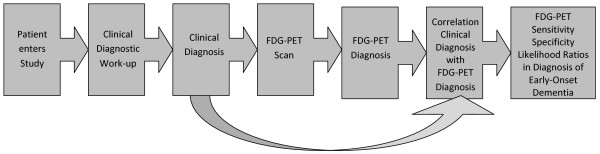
Study Design FDG-PET in diagnosis of early-onset dementia.
